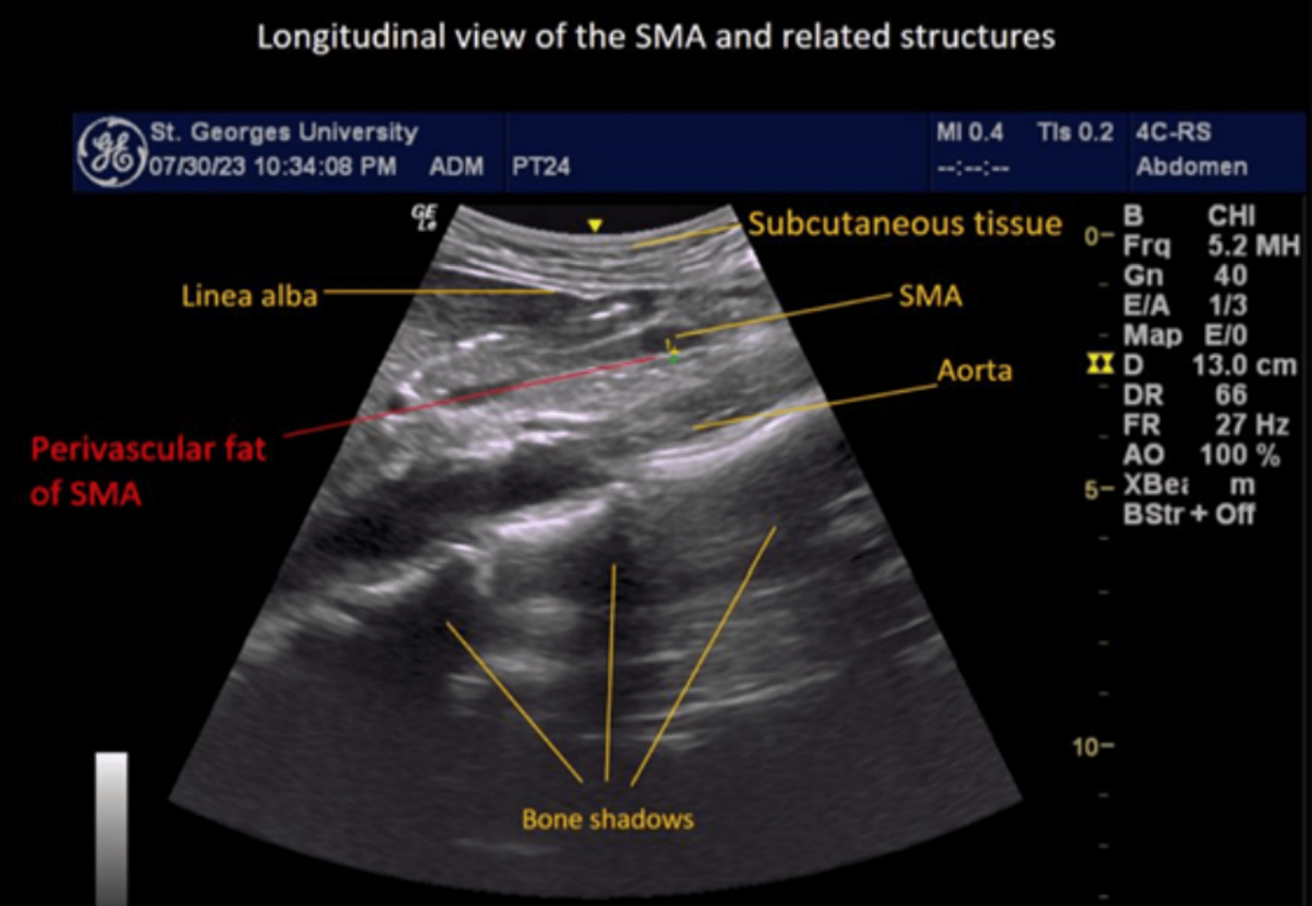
Ultrasound Image of the SMA and Surrounding Structures Ultrasound image of the SMA and its surrounding structures. The image highlights the subcutaneous tissue, linea alba, SMA, PVAT of the SMA, the aorta, and bone shadows. These components are clearly visible, providing a detailed depiction of the SMA and its associated PVAT. This image aids in understanding the SMA's anatomy and its relationship with nearby structures. SMA, superior mesenteric artery
